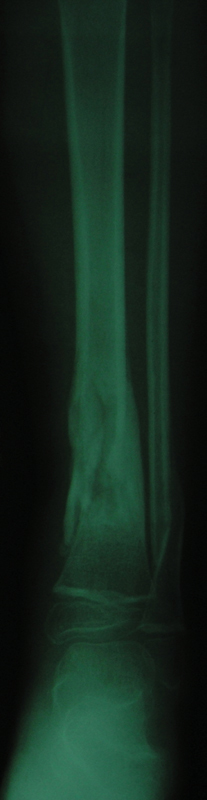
Anteroposterior roentgenogram of the left leg 4 months postinjury demonstrates complete union of the fracture in appropriate alignment. The extruded fragment was incorporated into the callus of the fracture site.
